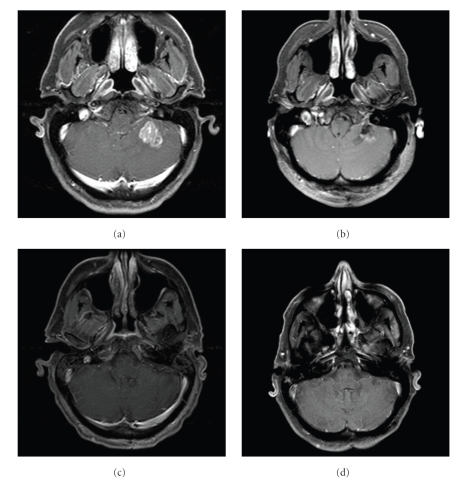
Axial, T1-weighted, postcontrast MR images of the brain. (a) A 2.3 × 2.3 × 2.5 cm left cerebellar lesion occurred synchronous with the diagnosis of a primary NSCLC. (b) The left cerebellar lesion was completely removed through a left suboccipital craniectomy. (c) No residual enhancement is noted after WBRT. (d) There is no evidence of recurrent brain metastasis on MRI obtained 2 years after diagnosis.
